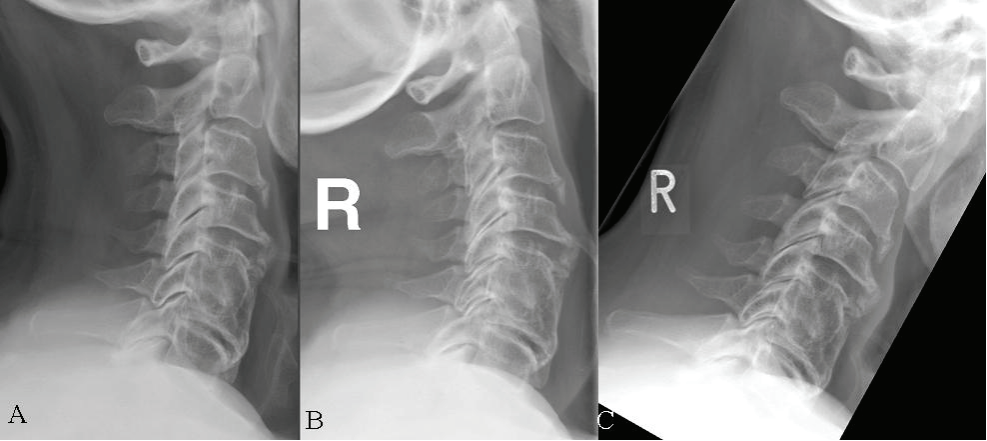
Exemplary plain (A), extension (B) and flexion (C) radiographs of a 67 years old patient with achieved fusion and ASD 7 years after surgery at level C5/6. In figure 1 a multisegmental degeneration can be seen.
